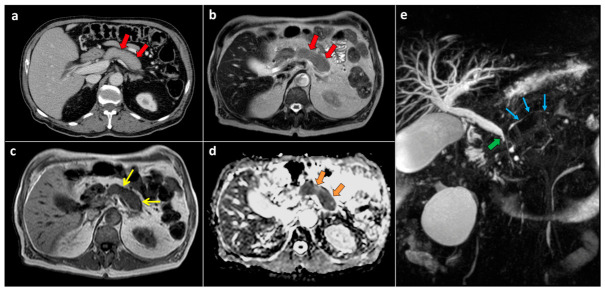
Diffuse AIP in a male patient presenting with painless jaundice and a cholestatic pattern. (a) Axial portal-phase CT shows a “sausage-shaped” pancreas with diffuse enlargement and loss of pancreatic clefts (red arrows). (b) Axial T2WI demonstrates a mild diffuse increase in pancreatic signal intensity (red arrows). The MPD is not clearly visible due to diffuse stenosis. (c) Axial T1WI shows a hypointense peripancreatic capsule-like “halo” (yellow arrows). (d) ADC map reveals low values within the pancreatic parenchyma, consistent with restricted diffusion (orange arrows). (e) Coronal 3D MRCP with MIP reconstruction demonstrates skipped, smoothly tapered “icicle-shaped” strictures of the MPD (blue arrows), associated with stenosis of the distal CBD (green arrow) and moderate upstream biliary dilatation.
